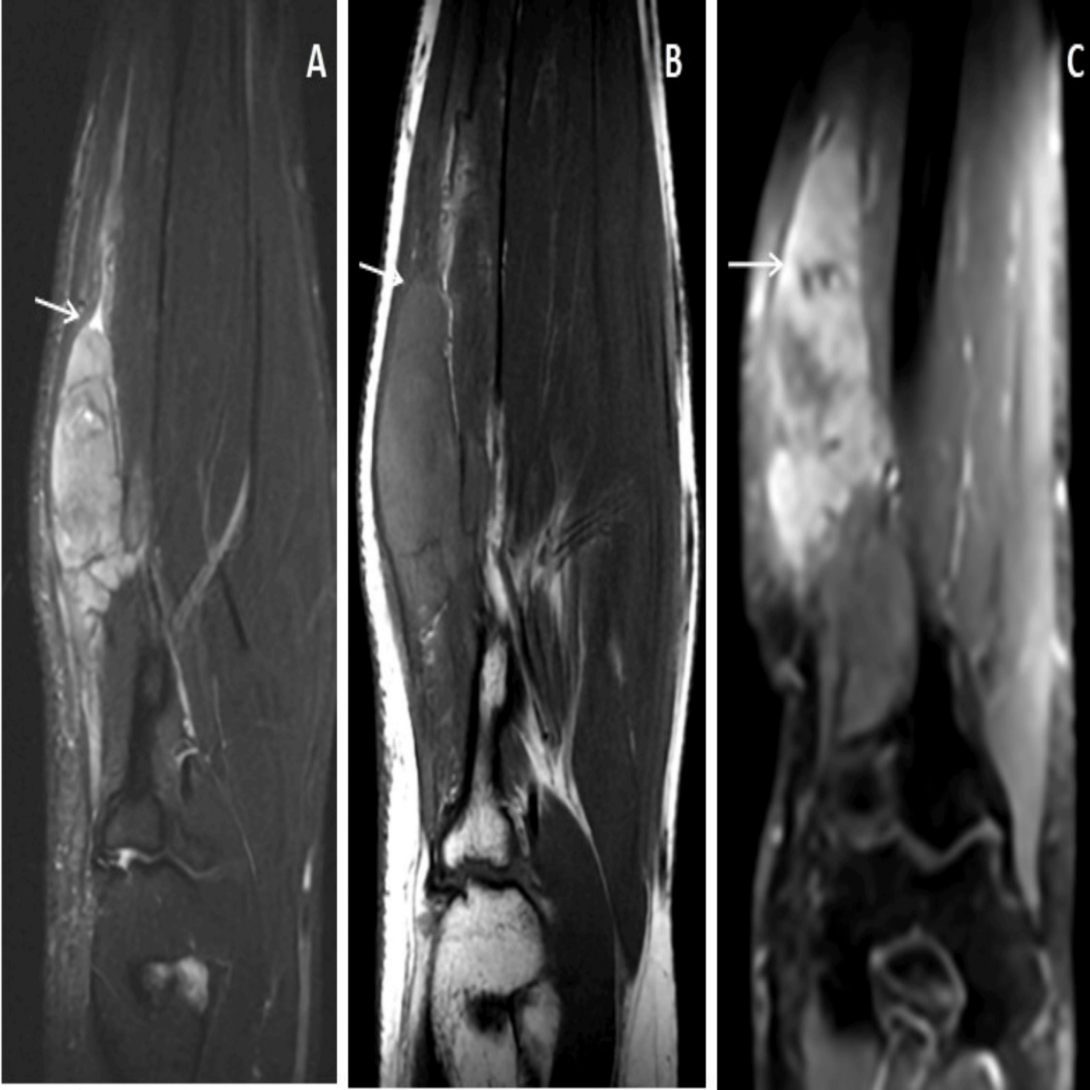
MRI images of the right forearm of the patient. The coronal proton density fat saturation (PDFS) and coronal T1-weighted (T1W) images (A and B) show a lobulated T1 isodense and PDFS hyperintense elongated appearing mass lesion seen in the radial aspect of the forearm infiltrating into the brachioradialis muscle. A positive fat sign is seen within the lesion (arrow in images A and B). On coronal post-contrast, the T1W image (C) shows the heterogeneously enhanching lesion with few non-enhancing cystic components.
